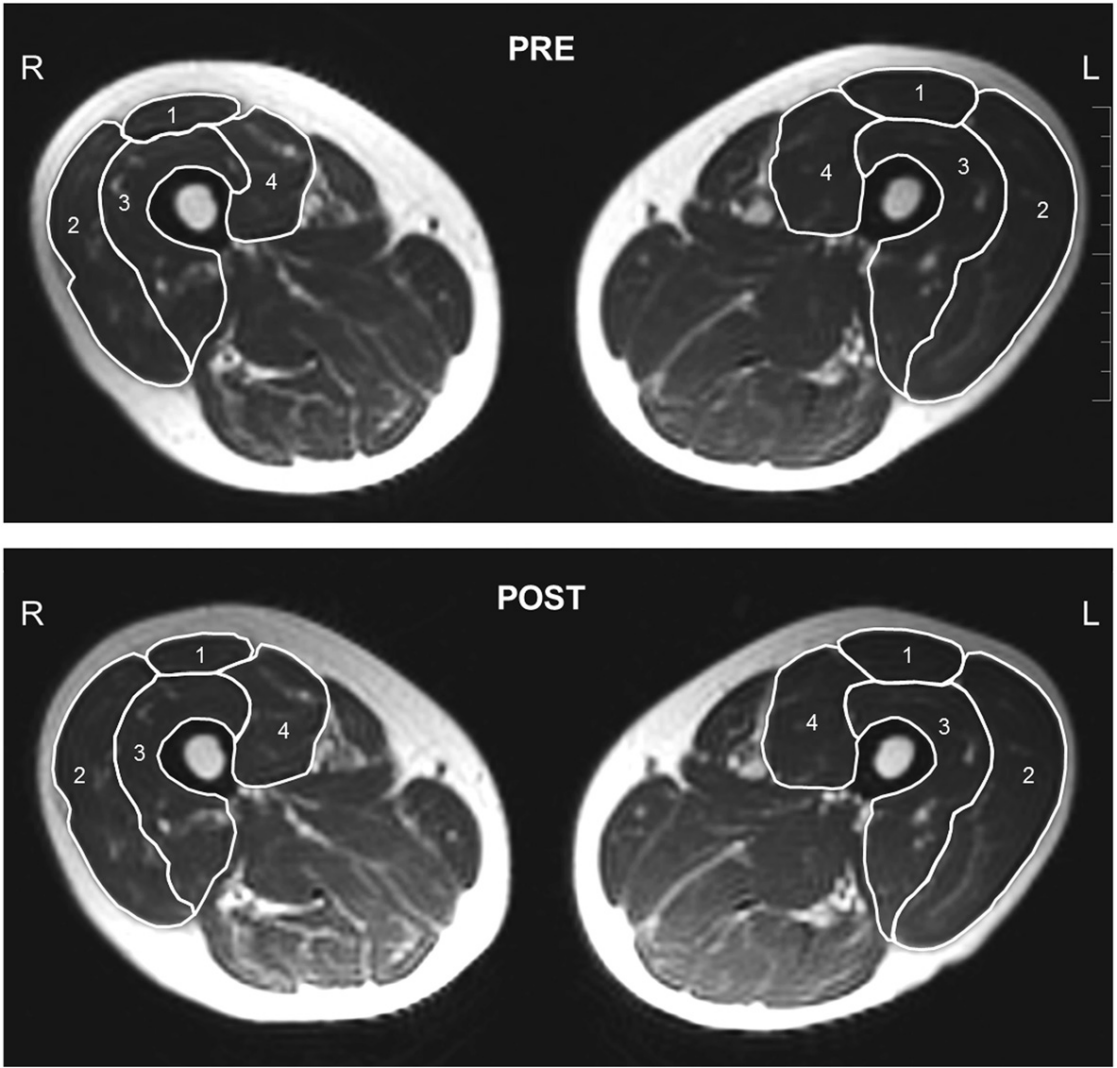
Magnetic resonance images showing thigh muscles (mid-thigh level) of more-affected (R) and less-affected (L) limbs of individual with stroke before (PRE) and after (POST) 12 weeks of ECC-overload flywheel RE training. Quadriceps femoris muscles denoted by numbers are: 1 m. rectus femoris, 2 m. vastus lateralis, 3 m. vastus intermedius, 4 m. vastus medialis. In this particular individual, m. quadriceps femoris volume of more-affected, trained limb increased by 11.8 %
